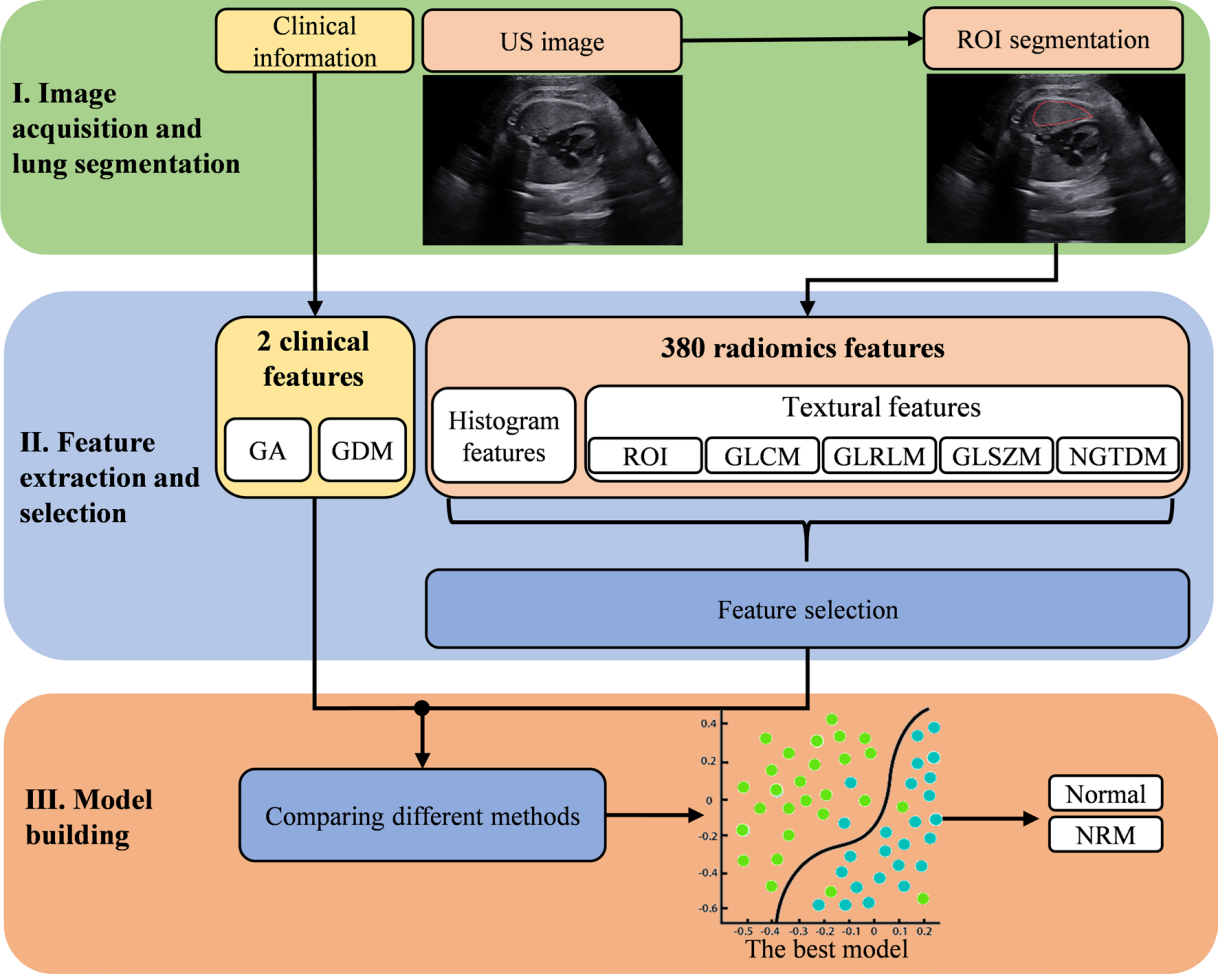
The Workflow of the entire study. Stage I: For each acquired fetal lung ultrasound image, the ROI inside the fetal lung is delineated by one physician and confirmed by another physician. Stage II: 308 radiomics features are extracted in the ROI of each image. Feature selection is performed on these radiomics features to select the most useful features. Stage III: the selected radiomics features are combined with the clinical features as the input to the classifier. With building and comparing classification models with different methods, the best model is finally selected to predict the risk value of NRM
